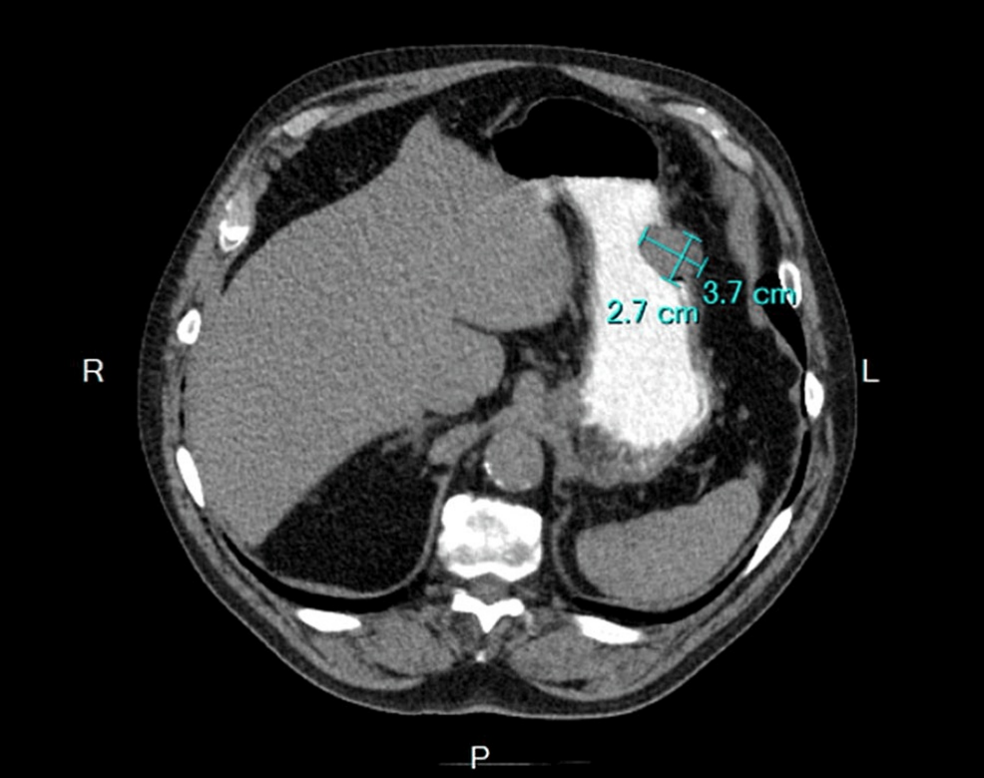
Pre-operative axial CT of the patient. Pre-operative axial CT of the patient showed a 3.7x2.7 cm fairly circumscribed intramural mass in the greater curvature of the stomach; no other abdominal masses were seen.
